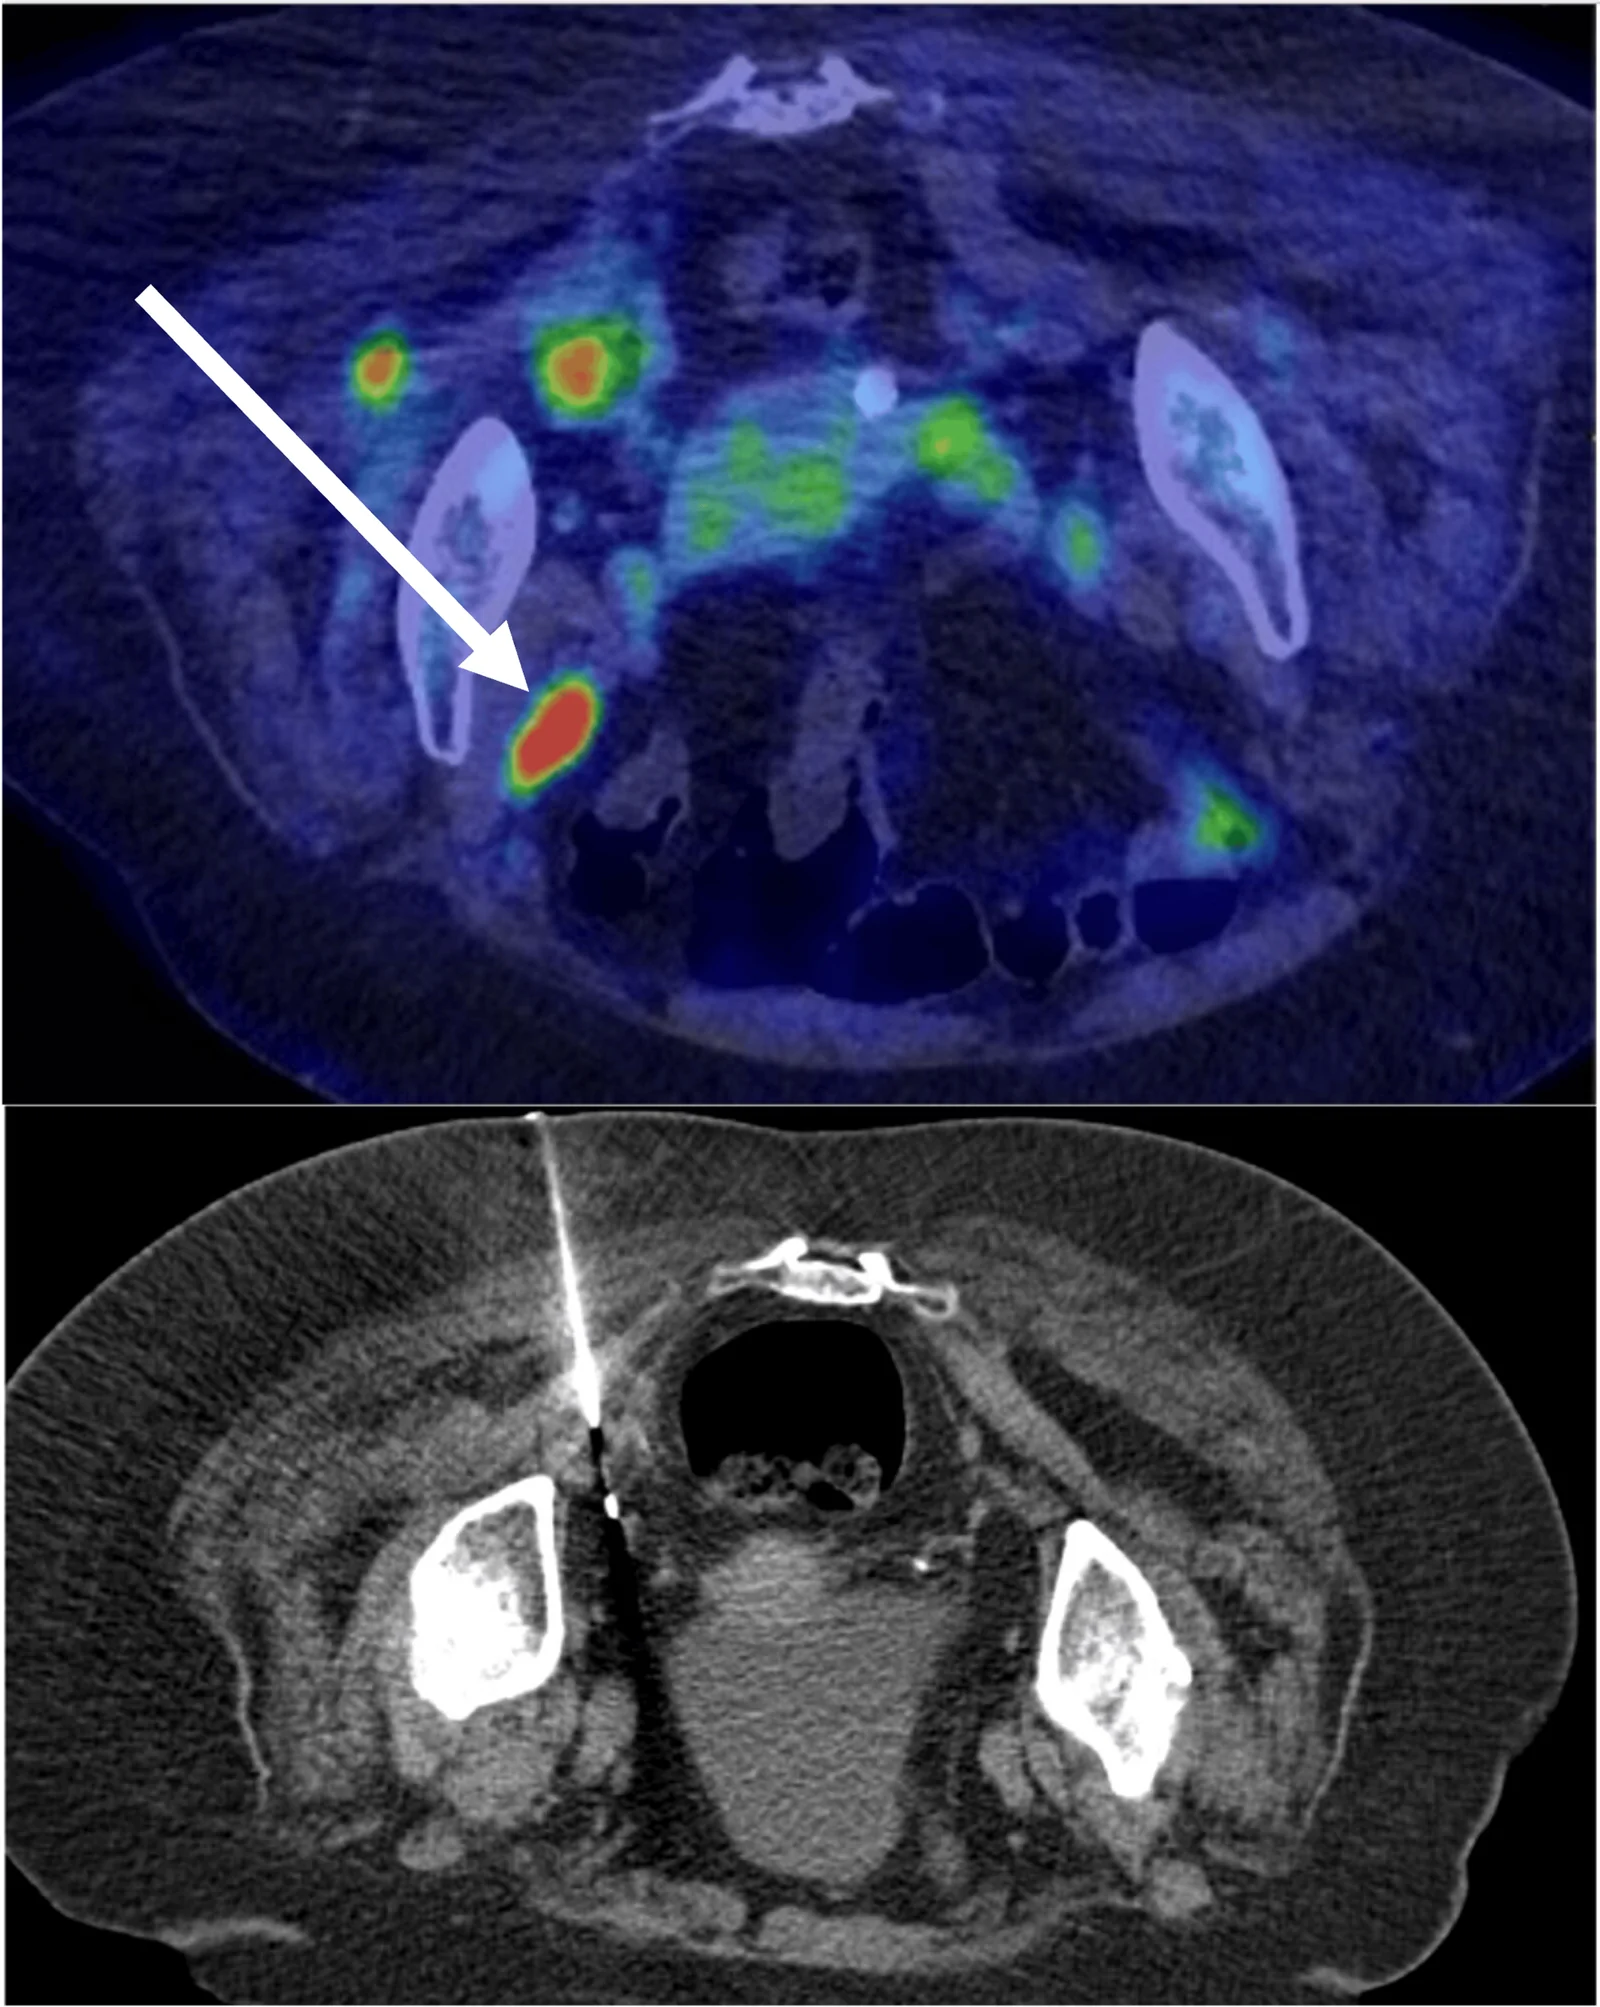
Above: FDG PET-CT slice at the level of the greater sciatic notch, demonstrating radiotracer avid uptake involving the right sciatic nerve (arrow). Below: CT-guided biopsy of the right sciatic nerve. FDG PET-CT = fluorodeoxyglucose positron emission tomography-computed tomography
